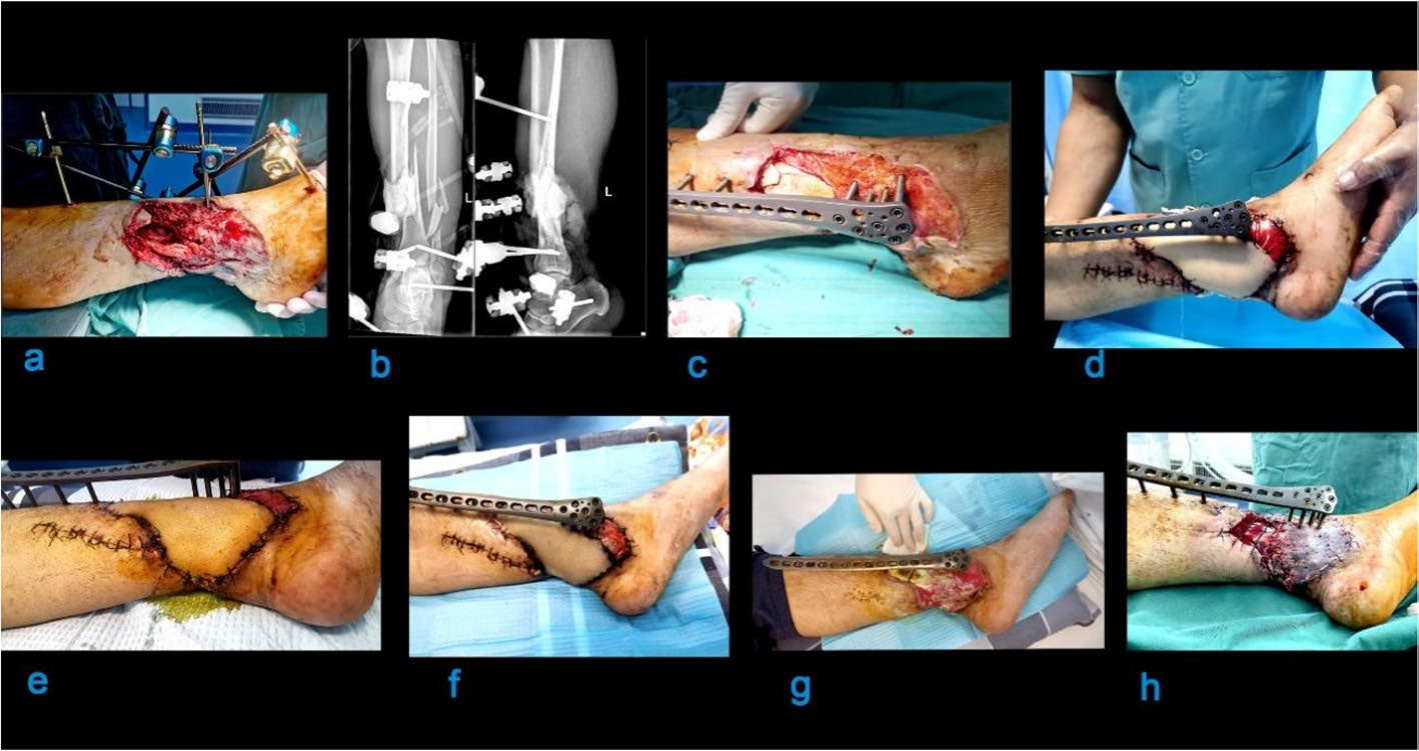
A 44 years old male patient with comminuted fracture (a) preoperative, (b) a computed tomography before the external fixator was replaced with the locking plate external fixator, (c) after repeated debridement, the external fixator was replaced with a locking plate external fixator, and the infection was controlled after VSD continues negative pressure drainage, (d) day one post-operation, (e) the 3rd-day post-operation, (f) sixth-day post-operation, (g) after re-vascular exploration, the skin flap gradually become necrotic, skin grafting was again performed, and repaired the local adjacent skin flap, (h) two weeks after the skin grafting and skin flap operation, the skin grafting area survive, with satisfactory blood circulation around the skin flap area
